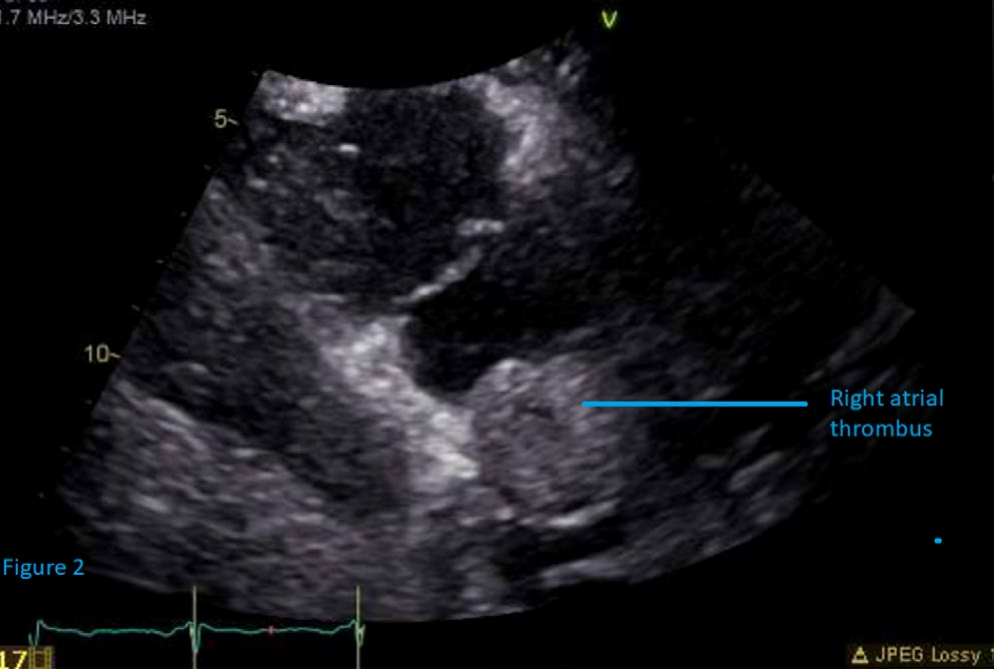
Echocardiogram showing right atrial thrombus (Blue line)
